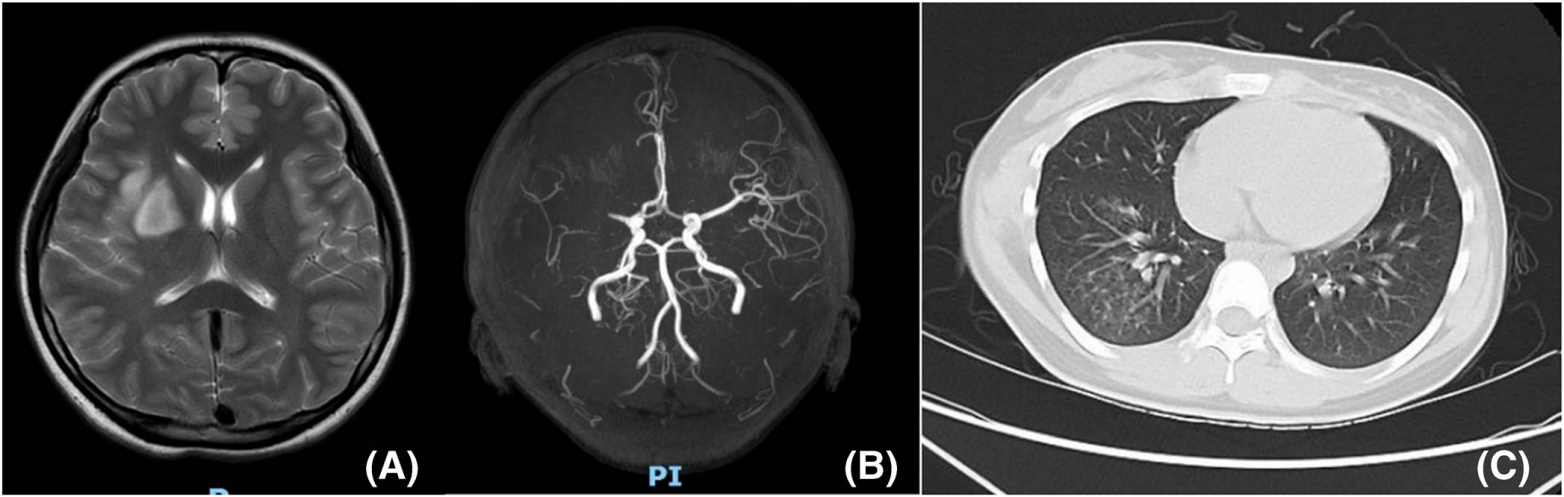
(A) MRI TW2 showed a high single change in the right brain; (B) MRA showed right MCA infarction; (C) the CT showed pneumonia of the right lower lung.
